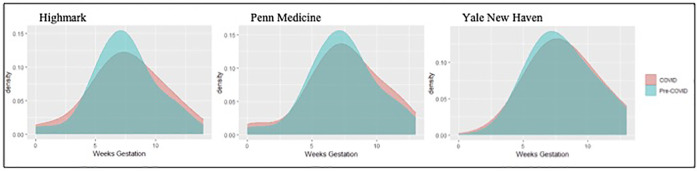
Distribution of completion of first trimester ultrasound across the three data sources.
